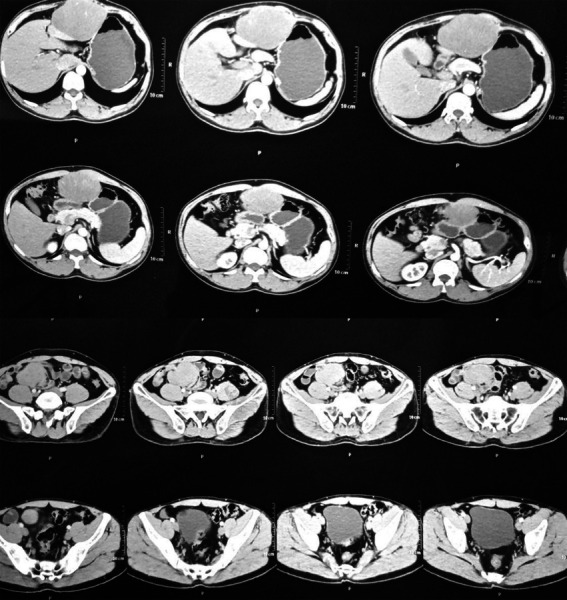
CT scan showing the described findings.
